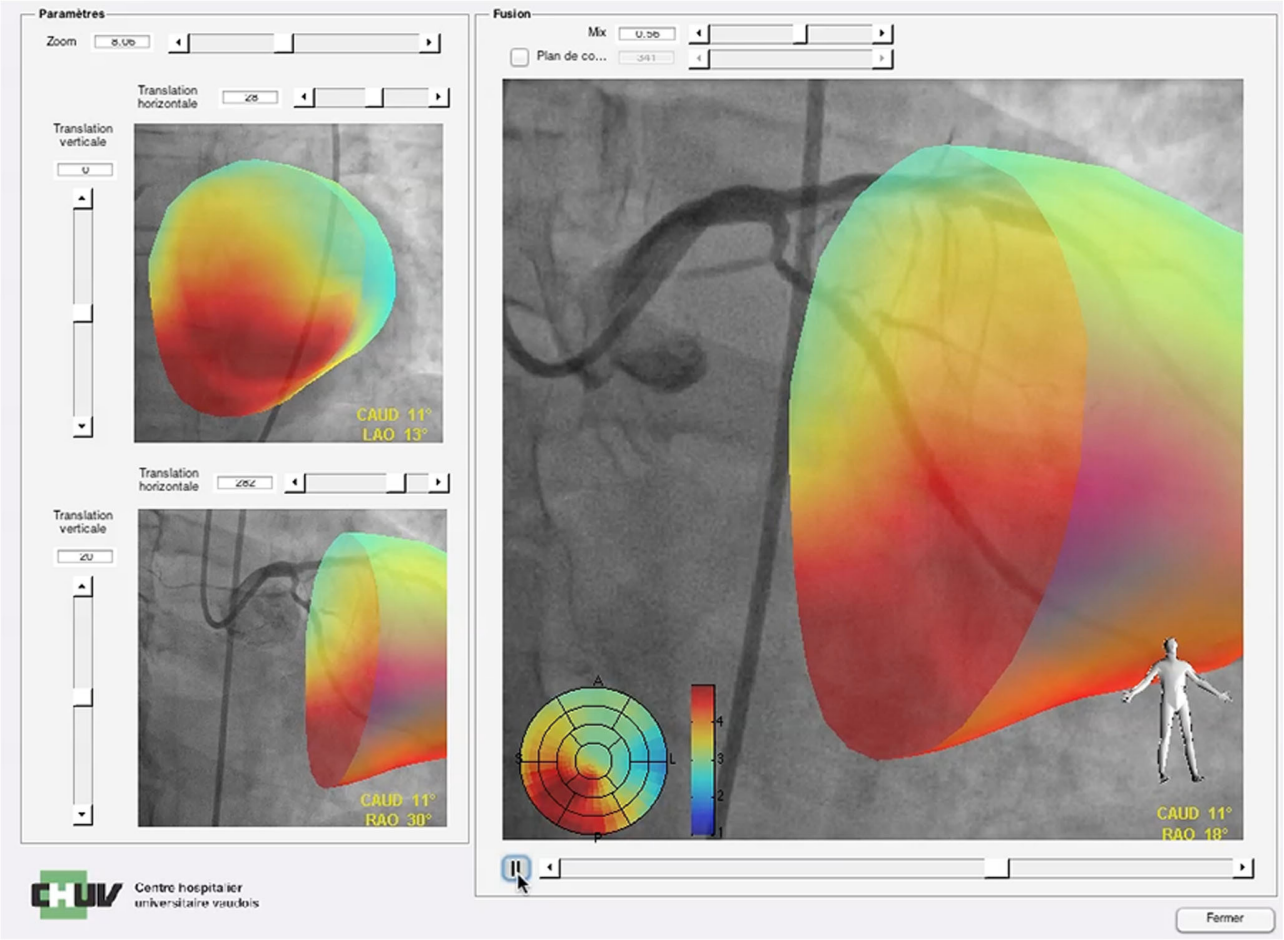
Example of PET/ICA hybrid imaging with our user interface allowing live fusion of PET myocardial blood flow information (in colour) onto angiographic projection of rotational X-ray angiograms (in black and white) in the catheterization laboratory
